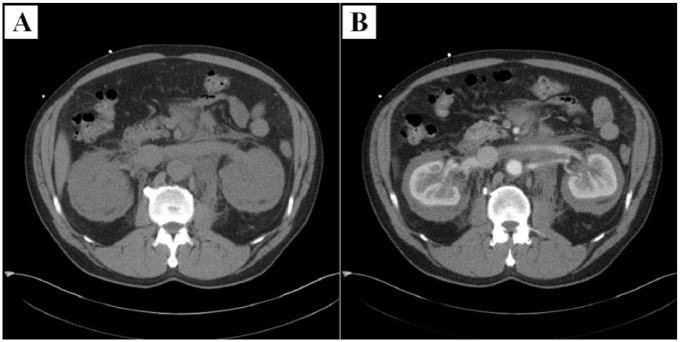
Abdomen computed tomography. Abdomen computed tomography showed an infiltrative soft tissue process in the abdomen surrounding the aorta, retroperitoneum, extending into the mesentery, and surrounding both kidneys (A). These findings mildly enhance after contrast administration (B) demonstrating the previously described “hairy kidneys” appearance in Erdheim-Chester disease.
